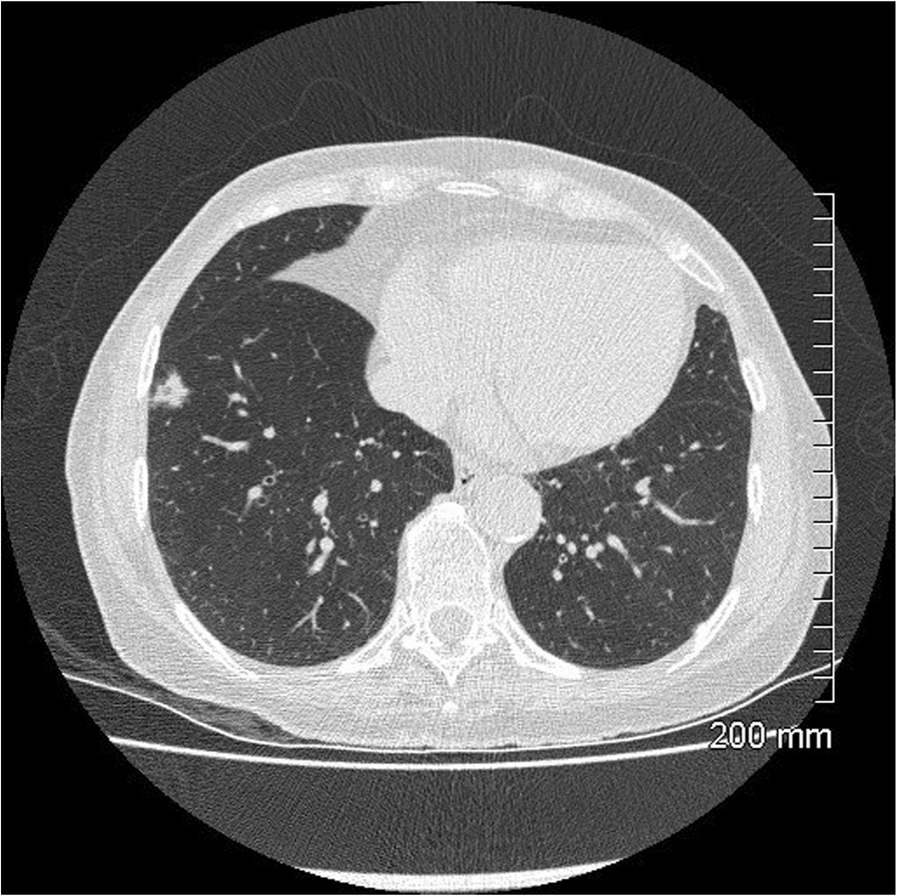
MIA on CT: 10 mm part-solid nodule, predominantly solid, in the right lower lobe
